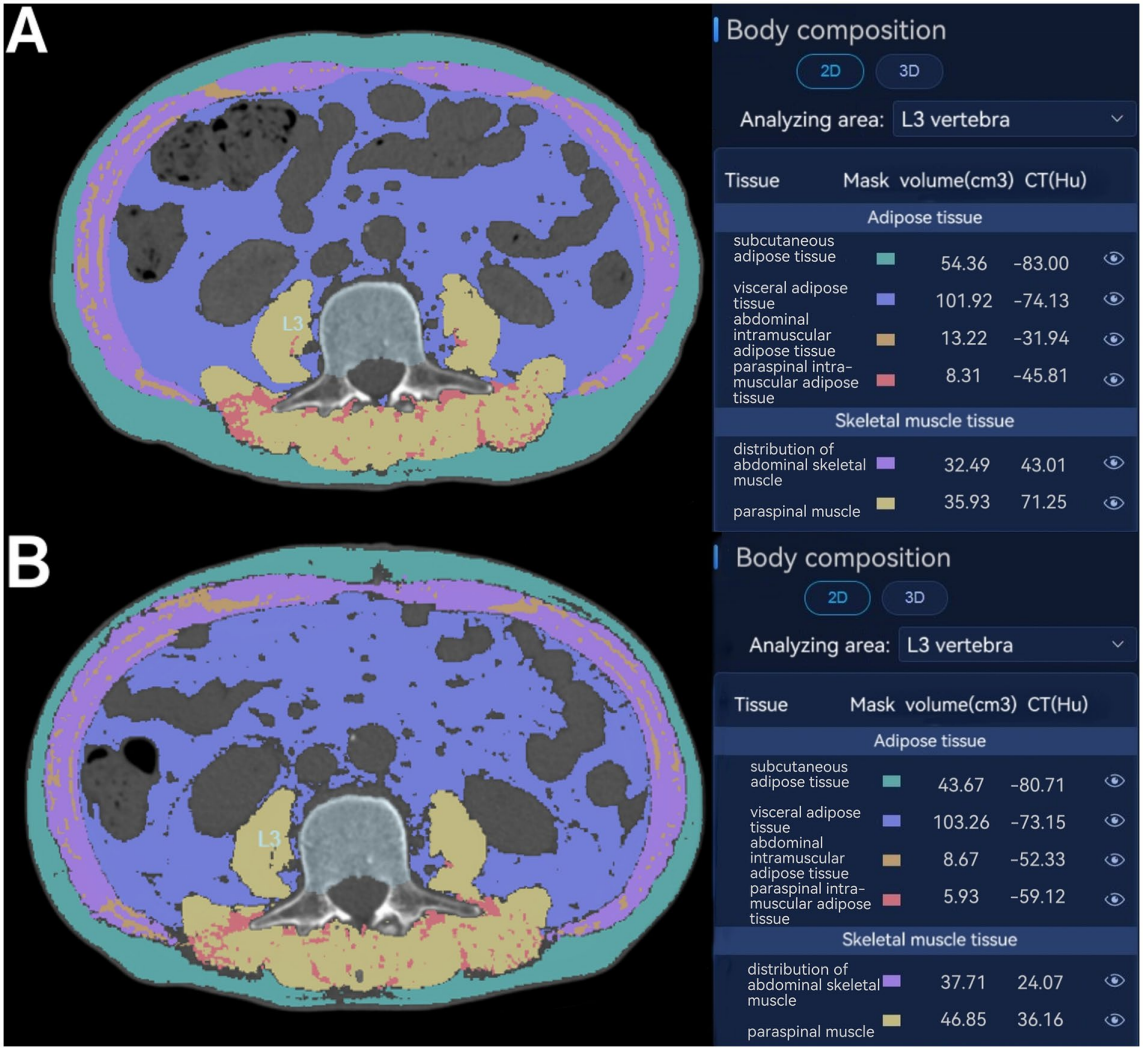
Assessment of body composition parameters on axial CT at the level of the inferior endplate of the third lumbar vertebra. Distribution of abdominal skeletal muscle (purple); abdominal intramuscular adipose tissue (brown); paraspinal muscle (yellow); paraspinal intramuscular adipose tissue (red); subcutaneous adipose tissue (green); visceral adipose tissue (blue) at CT before surgery (A) and postoperative day (POD) 7 (B).
